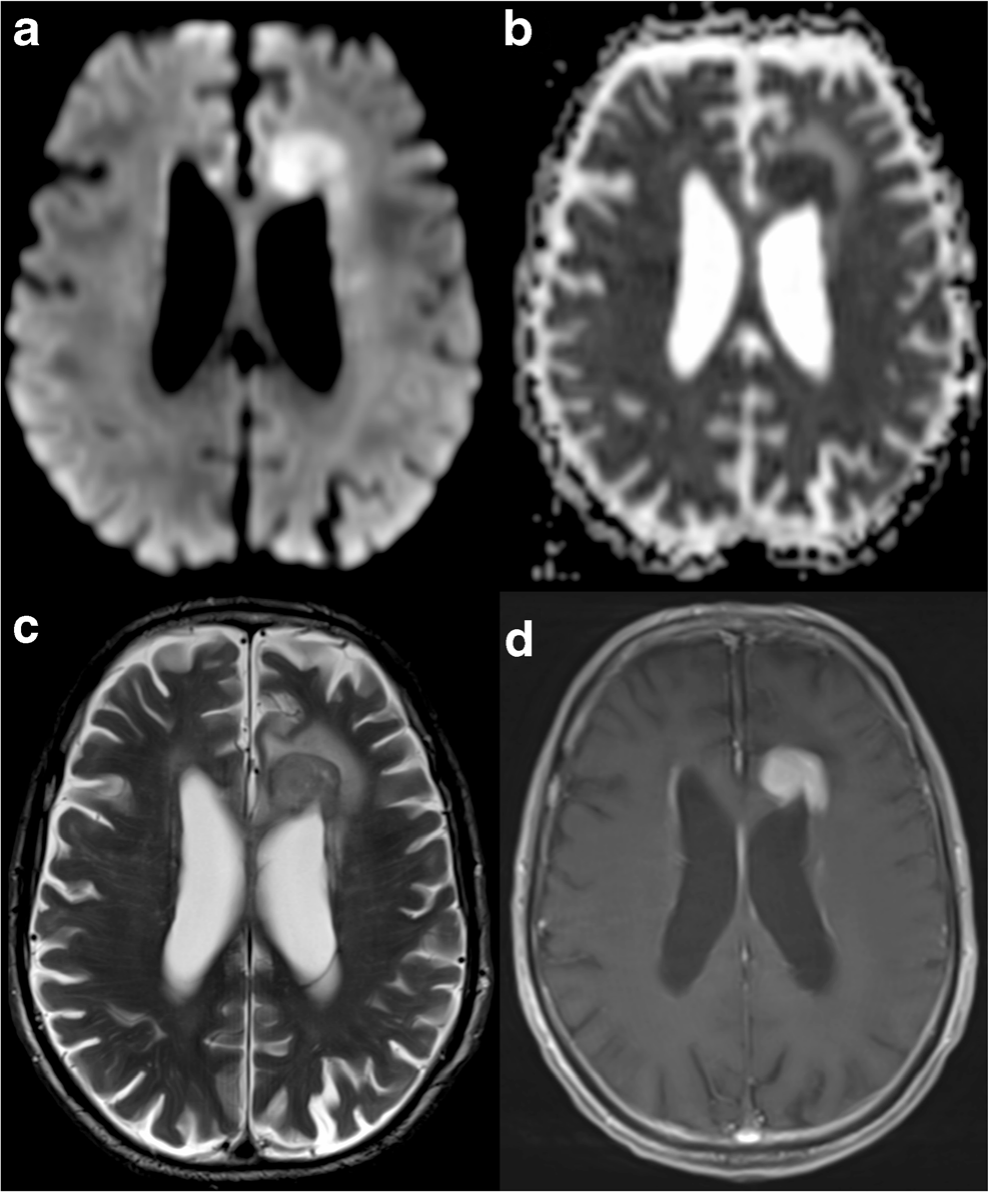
Primary CNS lymphoma. Left frontal periventricular lesion showing prominent diffusion restriction, presenting with hyperintensity on DWI (a), low ADC value (b) and mild hyperintensity on T2WI (c), all typical features of this type of hypercellular tumour. T1WI post gadolinium (d) shows homogeneous and intense contrast enhancement
